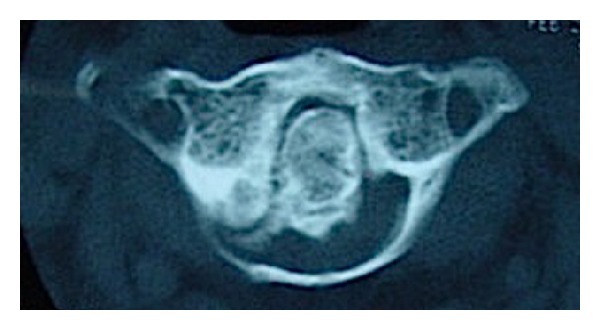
Axial CT scan at the level of the atlas demonstrating severe narrowing of the spinal canal and ossified transverse atlantal ligament.
